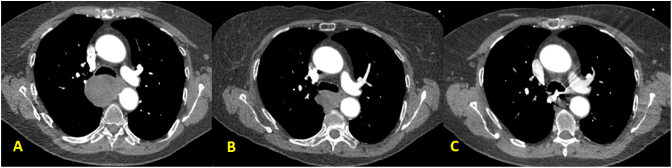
CT Angiogram showing regression of azygos vein aneurysm size at one-month 4.6 × 4.2 cm (A), three-months 3.3 × 2.3 cm (B), and six-months 1.0 × 2.4 cm (C) following endovascular treatment.
